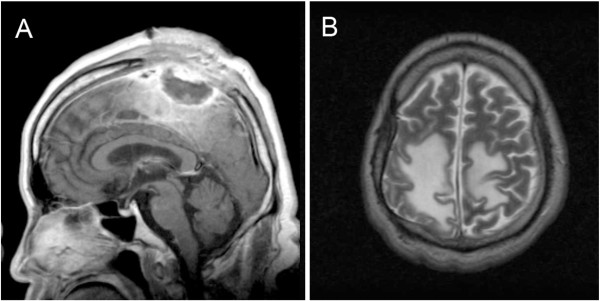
Images of the patient after the second procedure due to intracranial infection. Sagittal contrast-enhanced MRI scan (A) and axial T2-weighted image (B) prior to the third procedure of the infection demonstrating an irregular lesion on the apical lobe with massive hydrocephalus.
